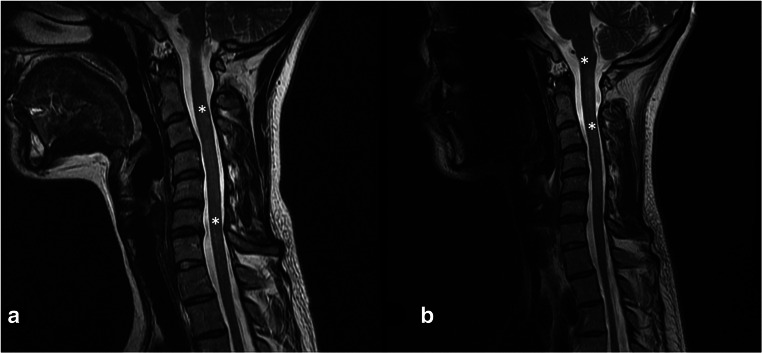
a Cervical spine MRI T2 sequences (May 2011). Diffuse hyperintensities of poorly defined borders, in C2–C3 down to the inferior plate of C5 vertebral body (asterisk). b Cervical spine MRI T2 sequences (2012). Hypertense image in in medulla-spinal border down to the inferior plate of C2 (asterisk)
